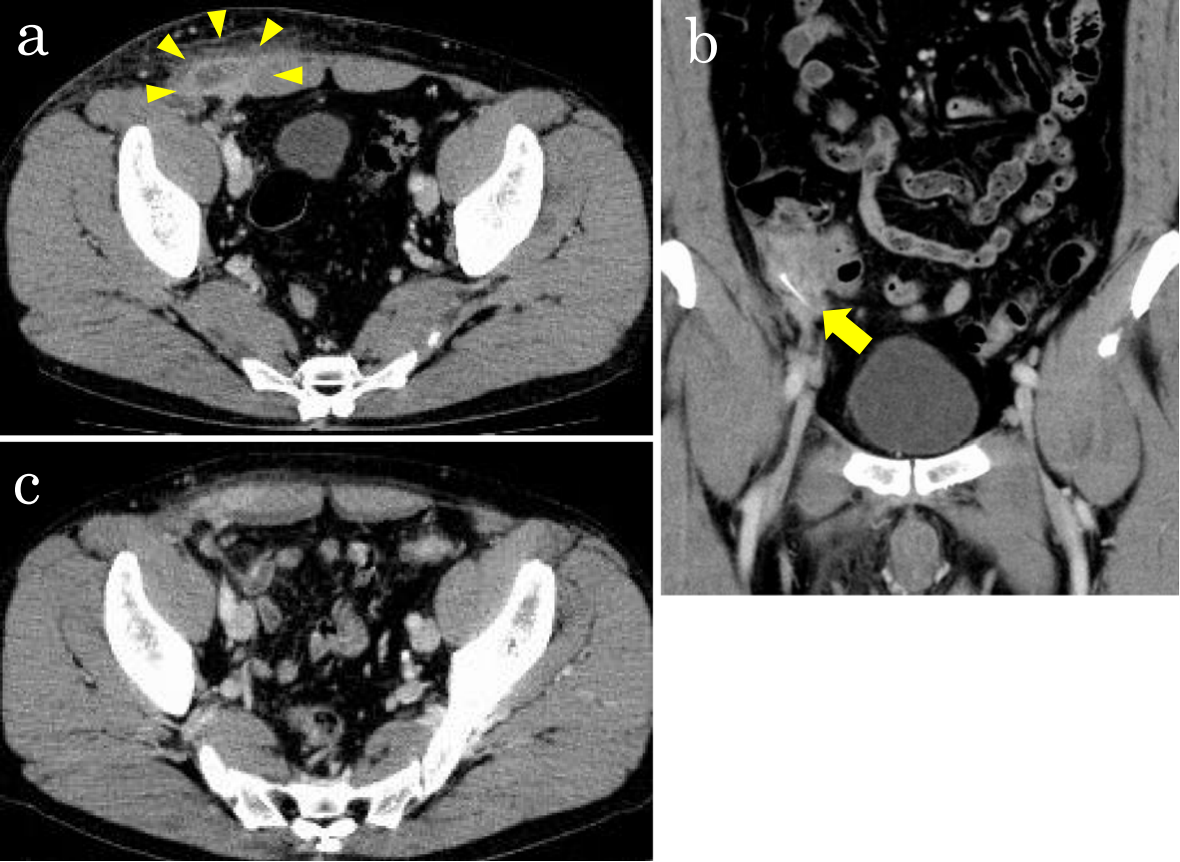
a Computed tomography scan shows a low-density area with rim enhancement in the right abdominal wall (arrowheads), and b a hyperdense, sharp object in the cecal wall adjacent to the low-density area (arrow). c After 2 weeks of antibiotic administration, the abscess is no longer apparent
